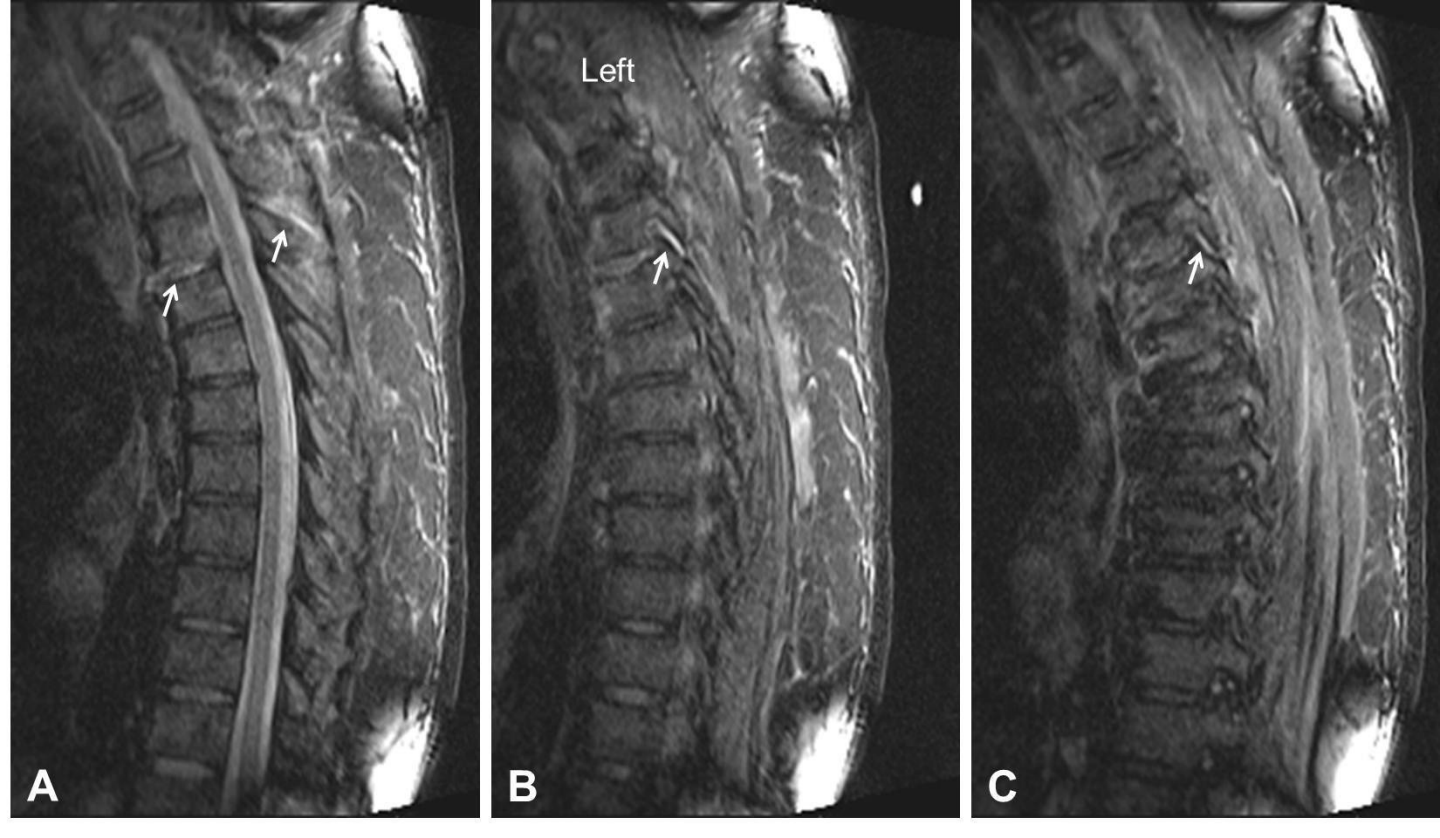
Magnetic Resonance Imaging (MRI) of Injury An MRI short tau inversion and recovery (STIR) sequence demonstrates high signal intensity at the level of the anterior longitudinal ligament, disk, and posterior ligamentous complex (interspinous and supraspinous ligaments) at the level of T4/5 indicating disruption (A, arrows). A left (B, arrow), and right (C, arrow) parasagittal image shows high signal intensity at the level of the facet joints indicating disruption.
